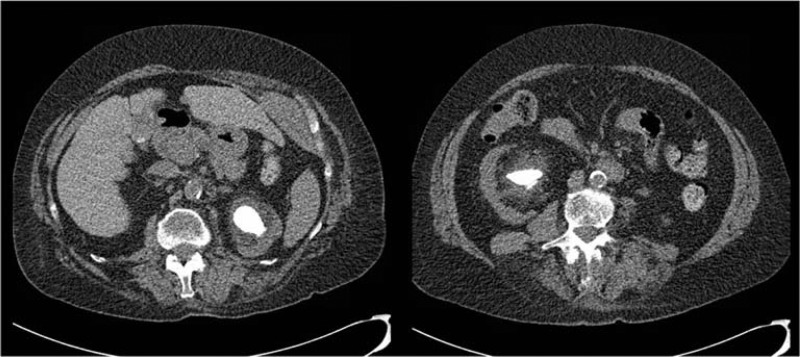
CT scan prior to left PCNL: Bilateral staghorn stones, left 3.2 cm renal staghorn involving 3 calyces, HU of 694, and right 3.4 cm renal staghorn involving 3 calyces, HU of 664. There is mild left hydronephrosis, bilateral parenchymal thinning and two left renal parenchymal calcifications (not shown).
